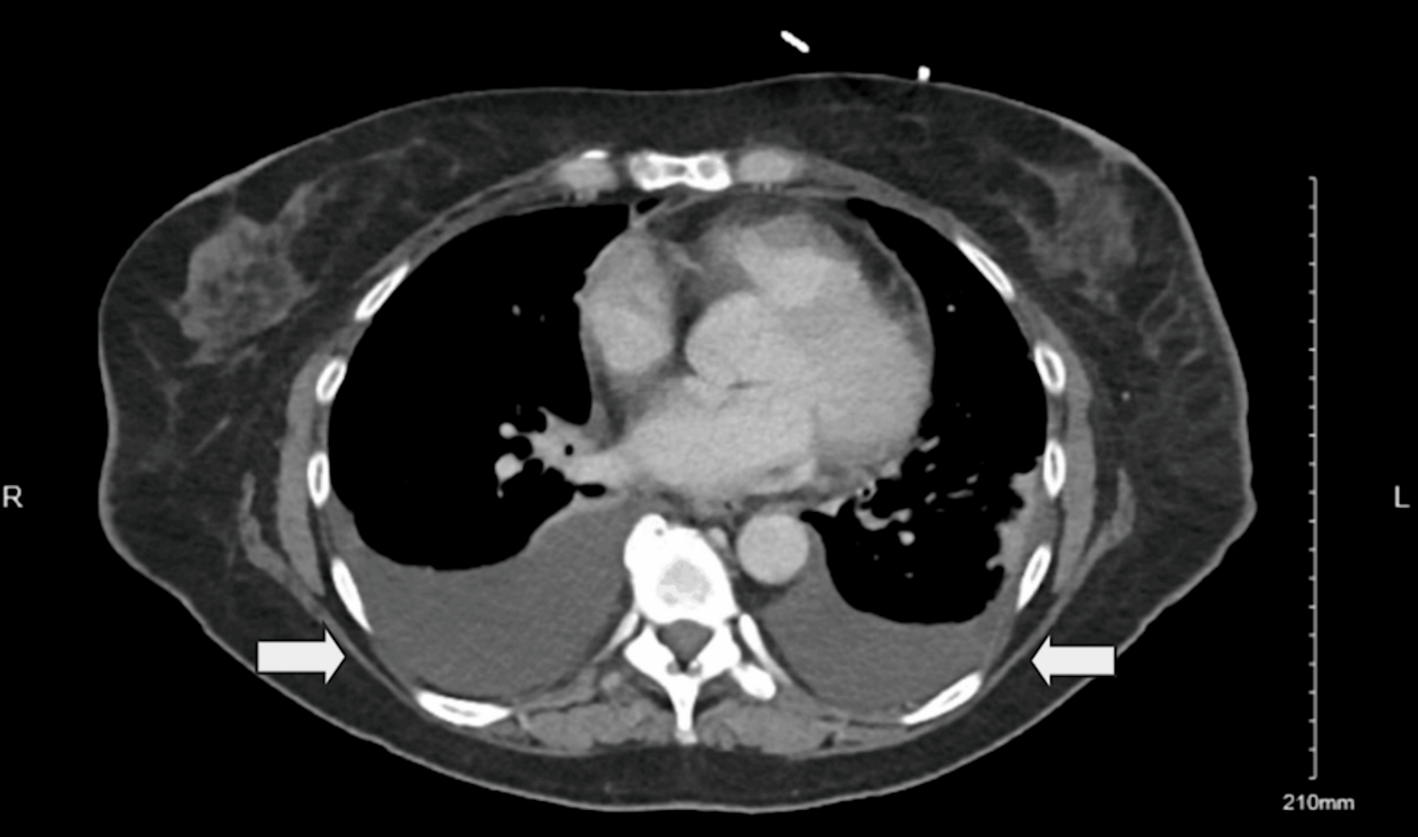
Chest computed tomography (CT) of the patient showing bilateral pleural effusion
